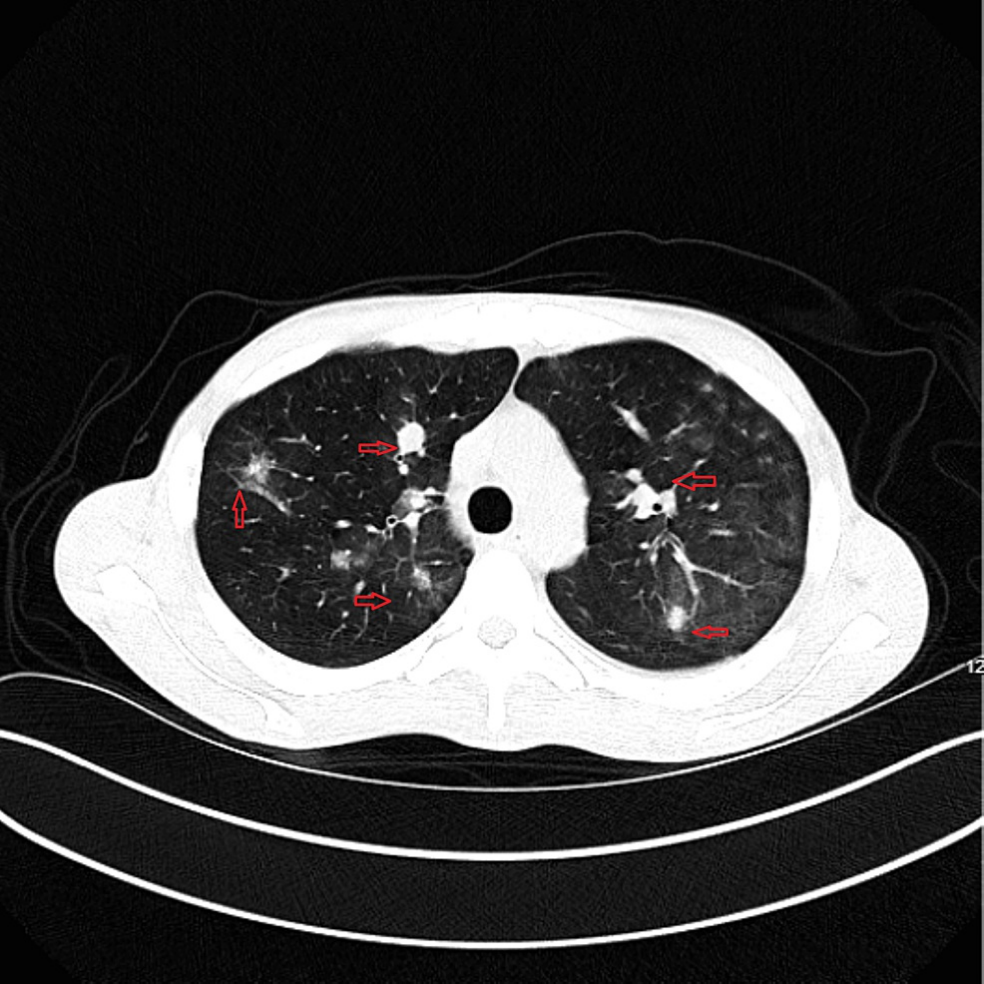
Computed tomography of the chest without contrast. The transverse section indicates ground-glass opacities and other lung lesions (red arrows).
